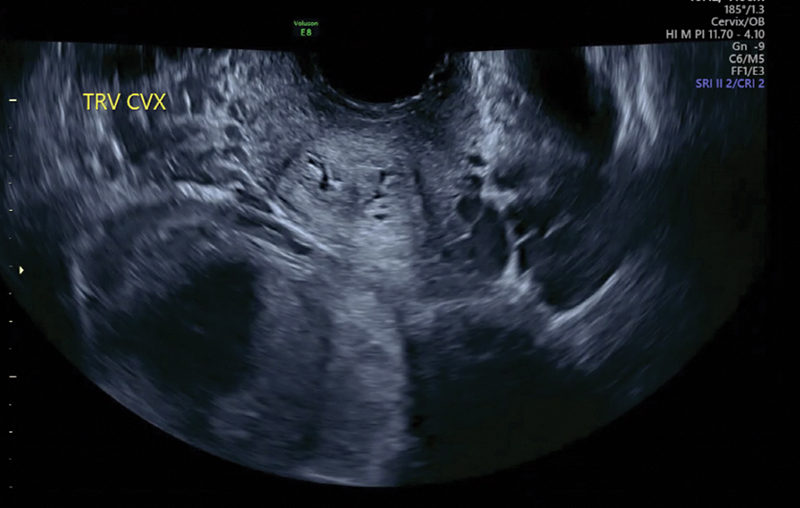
Transvaginal ultrasound at 18
